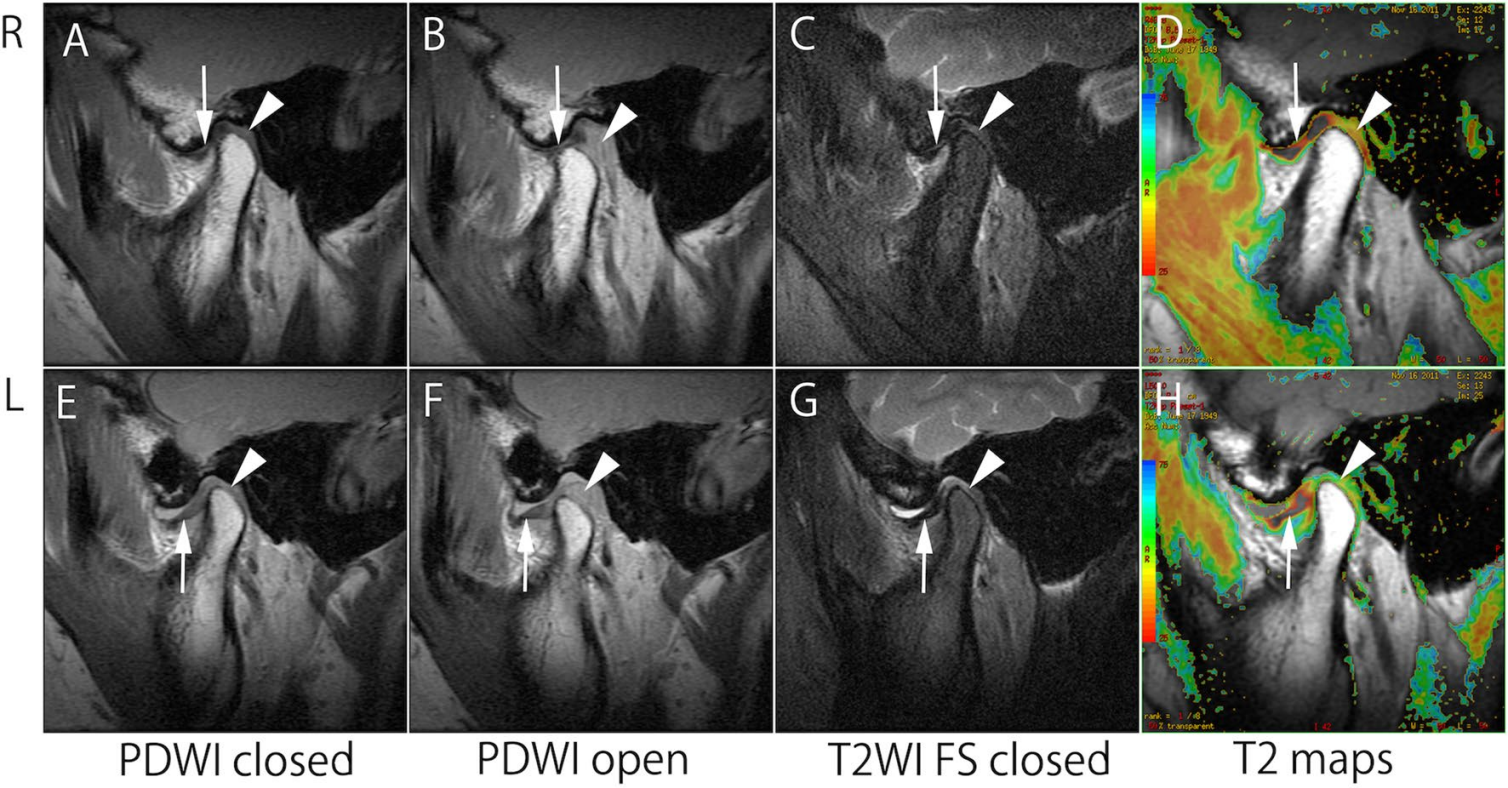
A 62-year-old female patient with unilateral ADDWOR and trismus. Right TMJ MR images (A–D) show normal superior disc position (NorSup) with normal function. The T2 values of the normal side were 25.8 ms for the articular disc (arrow) and 40.0 ms for retrodiscal tissue (arrowhead). Left TMJ MR images (E–H) show anterior disc displacement without reduction (ADDWOR) with joint effusion. The T2 values of the ADDWOR side were 29.1 ms for the articular disc and 51.0 ms for retrodiscal tissue.
